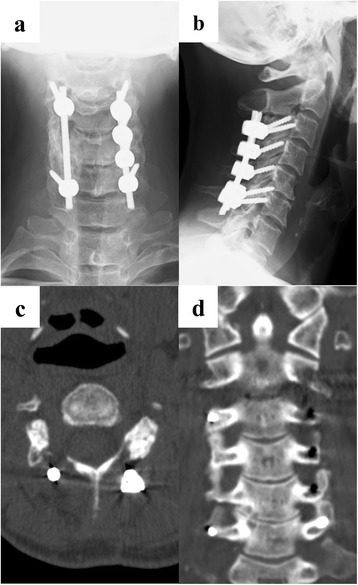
Two-year follow-up images. X-ray images show third-sixth cervical spine C3-C6 fusion and internal fixation: anteroposterior view (a), lateral view (b). Axial computed tomography scan image demonstrates disappearance of calcified mass in the intervertebral foramen of fourth-fifth cervical spine (c). Coronal computed tomography scan image also demonstrates disappearance of calcified mass at right side of spine (d)
